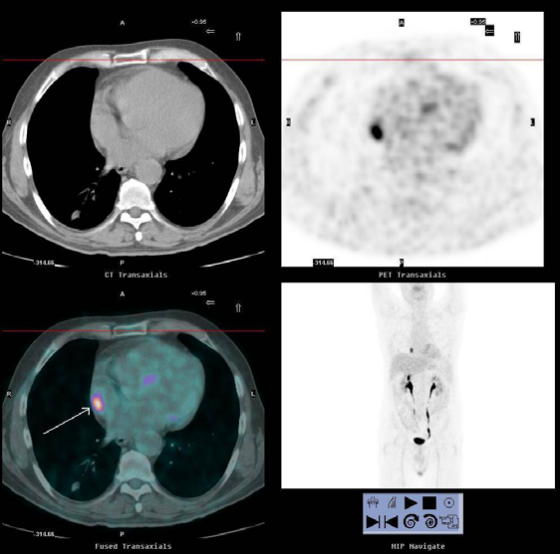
First [^18^F]FDG-PET/CT scan showing a suspicious uptake in the right atrium (SUV bw max 12).
